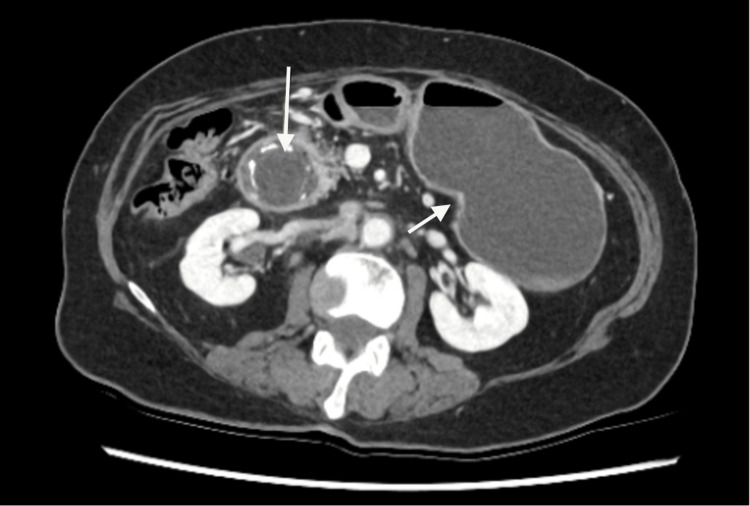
Axial slice CTAP with IV contrast demonstrating fluid filled distention of stomach and large obstructing 3 x 6 cm stone in D2 (Case 2) CTAP: computed tomography abdomen pelvis, IV: intravenous
